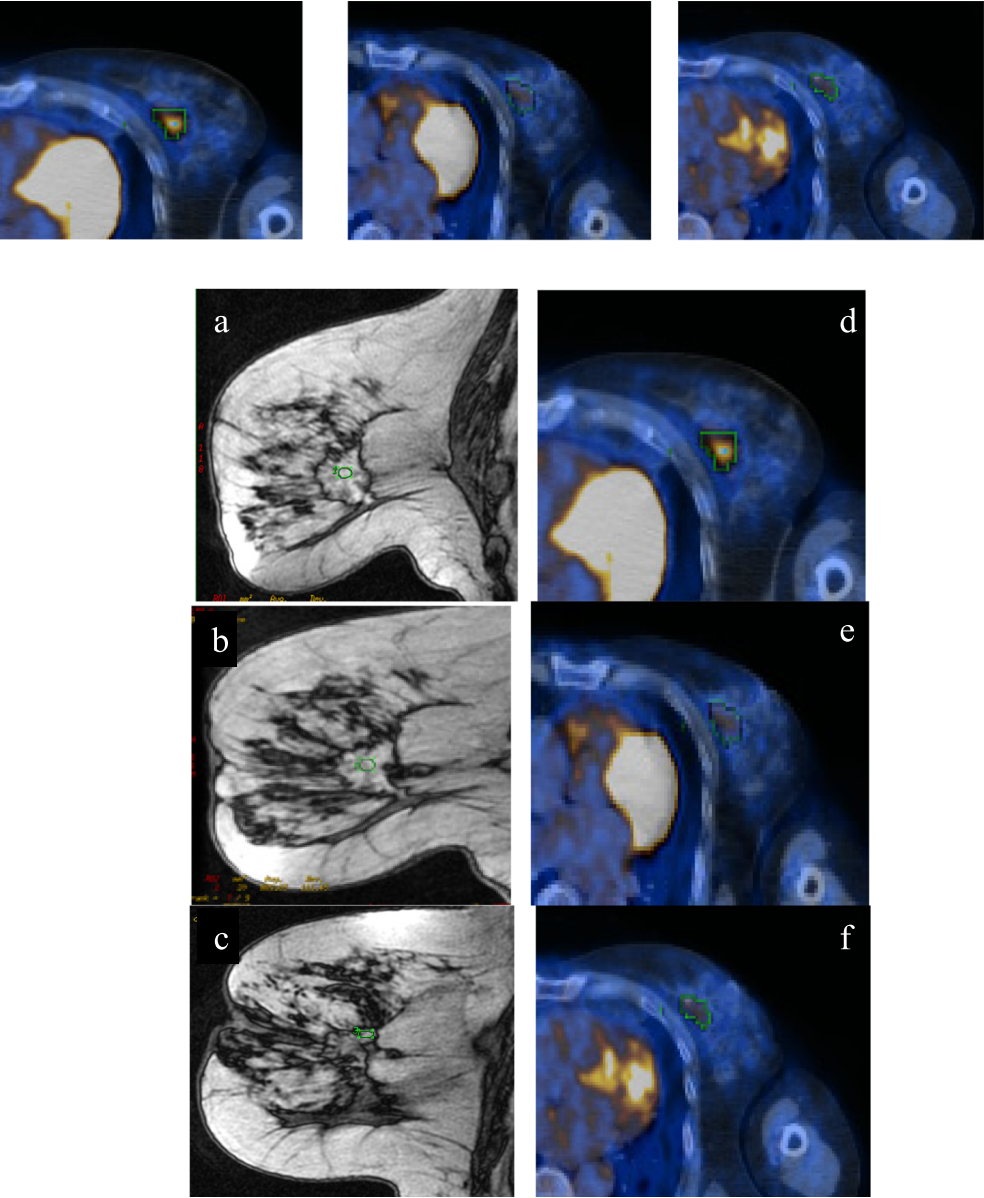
Decrease of tumor size on gadolinium-enhanced, T1-weighted sagittal MRI slices at M0 (a, 30 mm), M1 (b, 25 mm) and Pre-op (c, 22 mm) in a 76 year-old mR patient Metabolic response was demonstrated on FDG-PET/CT fused axial slices at M0 (d, SUVmax = 5), M1 (e, SUVmax = 3) and Pre-op (f, SUVmax = 3).
